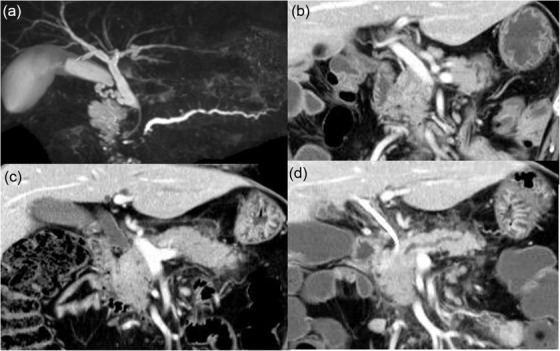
(a) Magnetic resonance cholangiopancreatography shows stenosis of the distal segments of the bile duct and main pancreatic duct and dilation of the upstream segments. (b) Coronal view of CT before the resection. (c) Coronal image of CT obtained 11 months after resection shows swelling of the entire pancreas. (d) CT image at the time of the second endoscopic ultrasound‐guided fine needle aspiration (EUS‐FNA) shows atrophic changes in the body and tail of the pancreas, peripancreatic soft tissue infiltration, and swelling of the head of the pancreas. *(a): After endoscopic biliary drainage. A plastic stent is placed in the common bile duct
